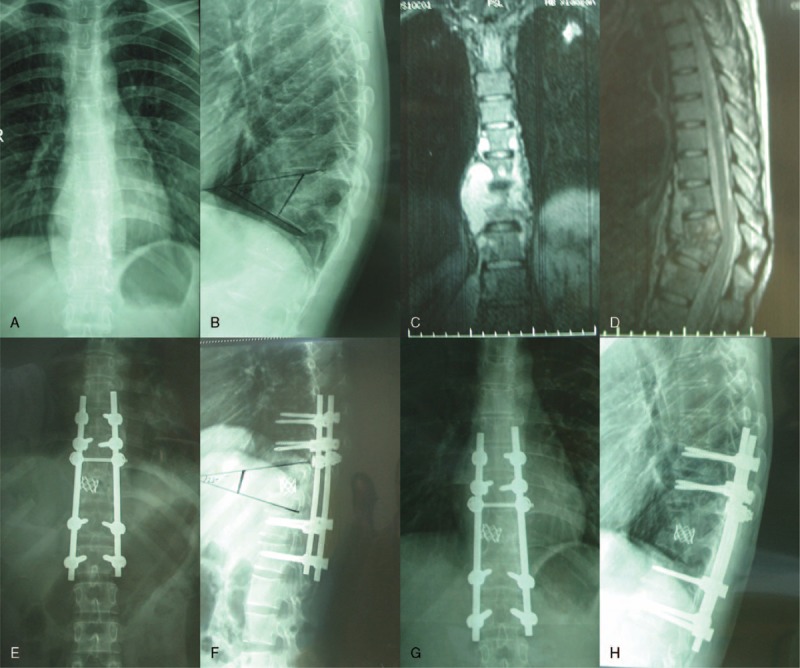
A 25-year-old female patient (a–h), preoperative X-ray (a, b) and MRI (c, d) shows T8, T9 vertebral body bone destruction with cyrtosis, compressed spinal dura mater, and paravertebral abscess. Postoperative 1 week, X-ray (e, f) show vertebral body height corrected with Cobb angle. Postoperative 1 year, X-ray (g, h) showed a good fixed position. MRI = magnetic resonance imaging
